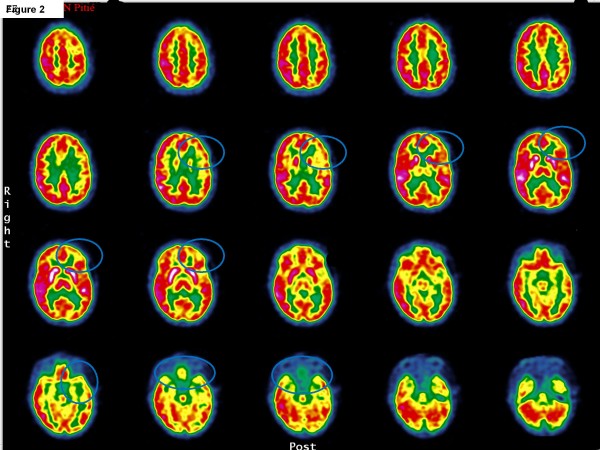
Left frontal-temporal cortex hypometabolism and moderate bilateral hippocampic hypometabolism in cerebral positron emission tomography (^18^FDG-PET).
